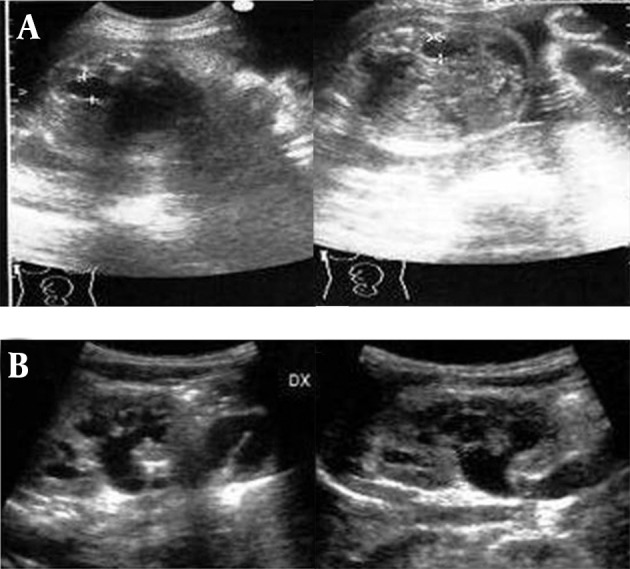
A. Fetal ultrasound at 31 weeks of gestational age demonstrating bilateral hydronephrosis grade 1 (SFU). B, Ultrasonography of the right and left kidney on admission showing bilateral ureterohydronephrosis grade 3 (SFU)
